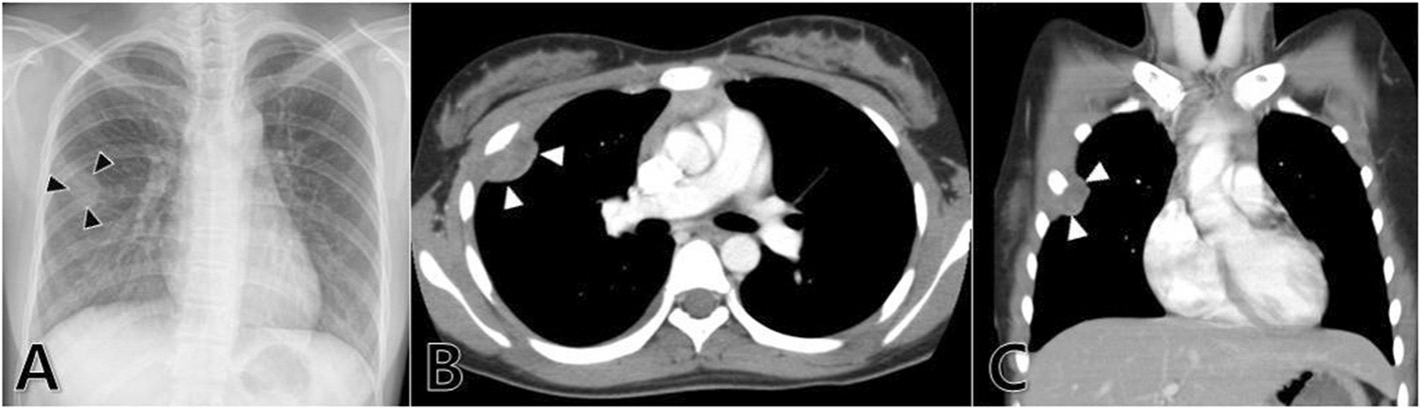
CT scan revealing a small mass with a broad base. A small nodular opacity (black arrowheads) in the right chest on chest radiograph (A) revealed a mass (2.7 × 2.2 cm in size) on the pleura of the right anterior hemithorax (white arrowheads) with irregular soft tissue density and inner heterogeneous enhancement on CT scan (B) and (C).
